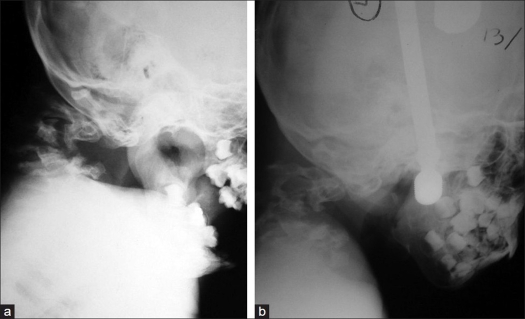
A 8-year-old boy presented with cervical kyphosis and inability to walk. Lateral radiograph of cervical spine (a) shows spondyloloptosis at C2/C3 with kyphotic angle between C2/4 of 120°. After skull traction, radiograph (b) shows some degree of kyphotic correction
